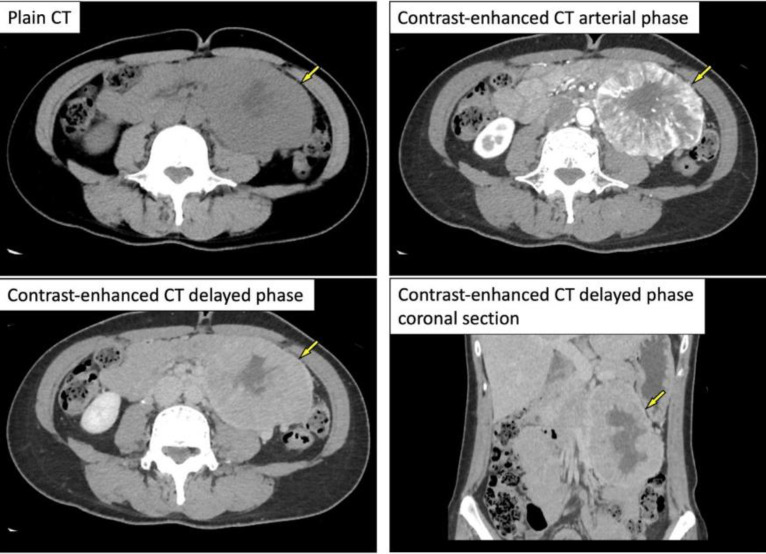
Contrast-enhanced computed tomography (CT) revealing an 8 × 7 × 12cm^3^ mass lesion in the left retroperitoneal space (⇨). Dynamic CT revealing high-contrast enhancement in the arterial phase and washout in the delayed phase
